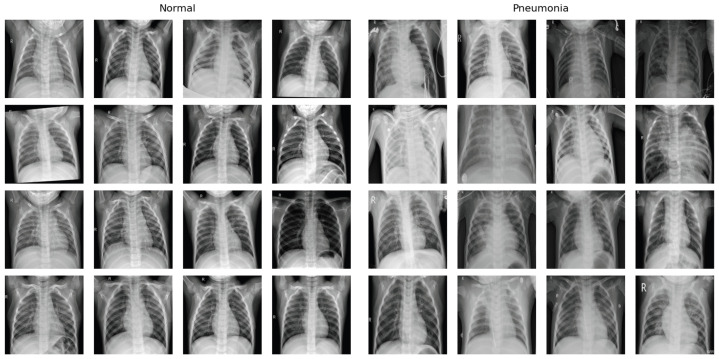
Example X-ray images of each class within the Pneumonia X-ray dataset. The images on the left showcase normal X-ray images and the images on the right showcase pneumonia images.
